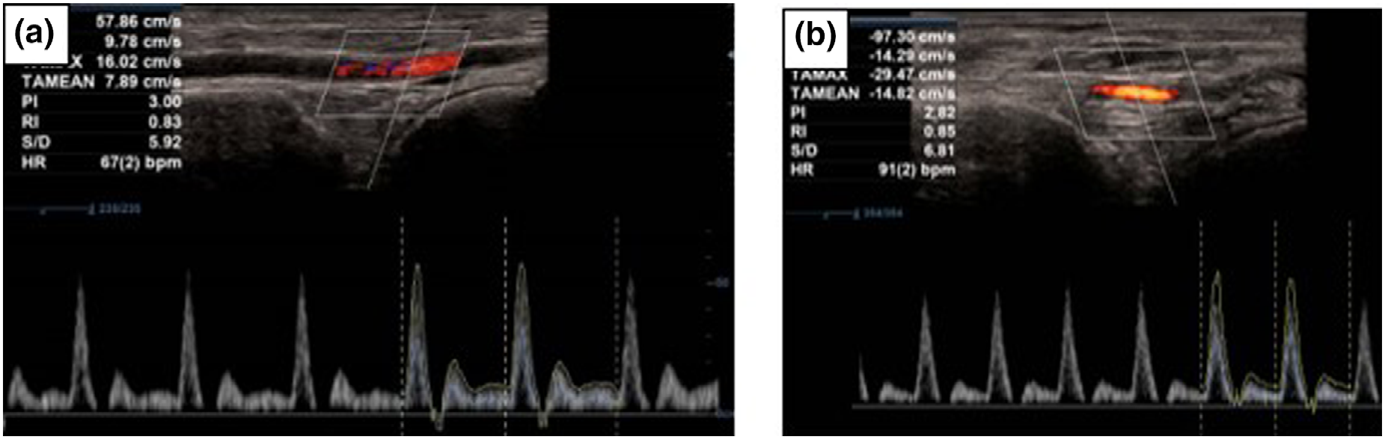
(a) Spectral trace of proximal dorsalis pedis artery for control participant; (b) Spectral trace of proximal dorsalis pedis artery for participant with rheumatoid arthritis. Normal multiwave Doppler velocity was observed for both participants.
